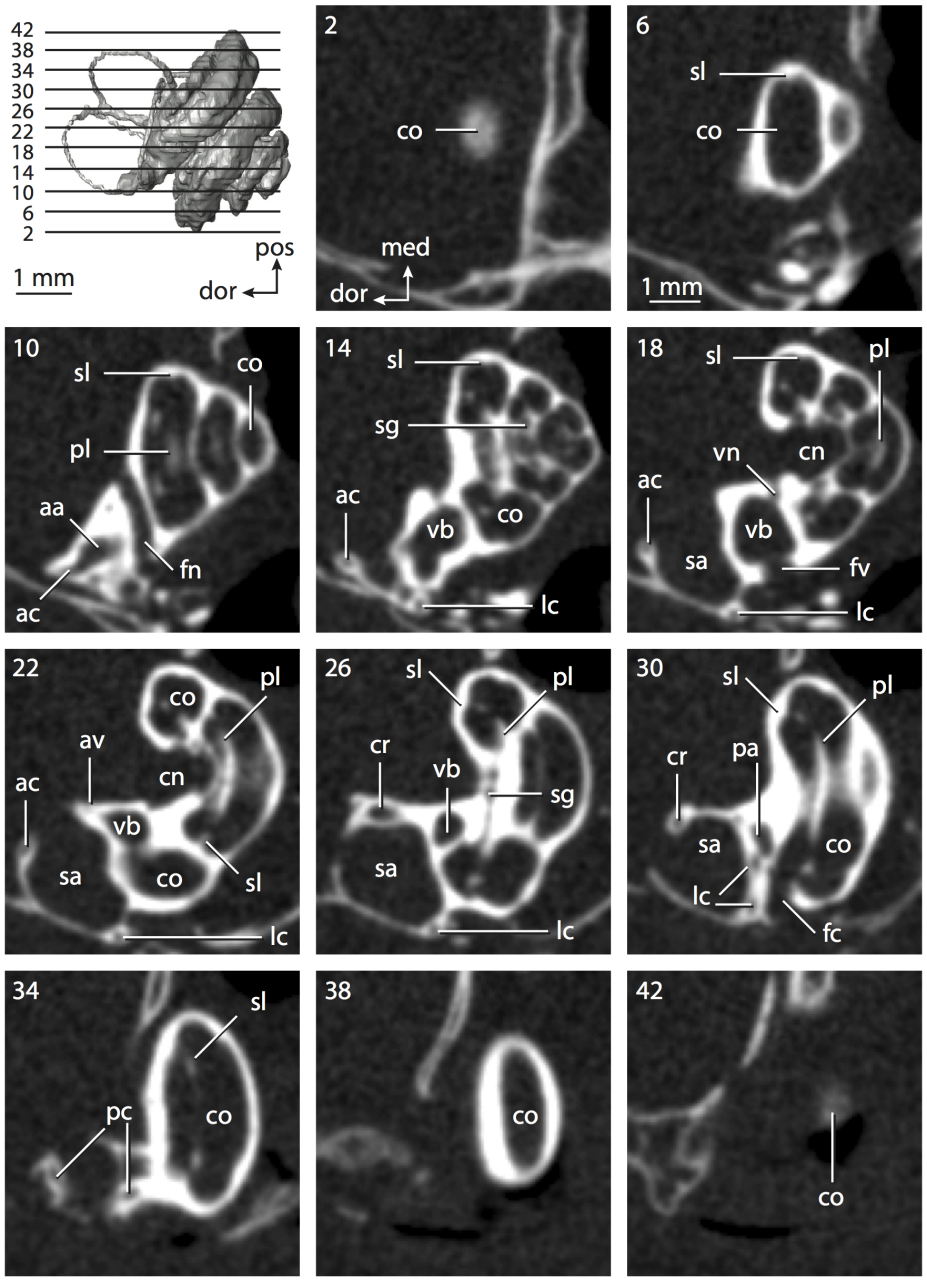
CT slices through ear region of*Rhinolophus ferrumequinum.* Abbreviations listed at the end of the Materials and Methods section.
